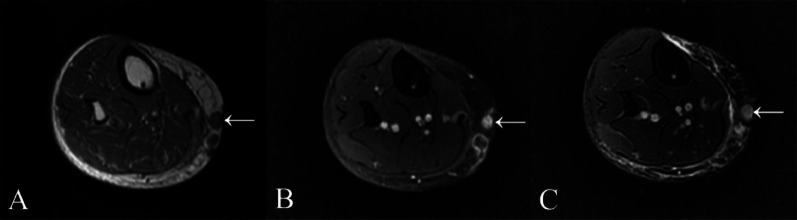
MRI: (A) Leiomyosarcoma appears hypointense on T1. (B) Hyperintensity on T1 + C. (C)T2 hyperintensity on fat-suppressed sequences. +C, contrast-enhanced
